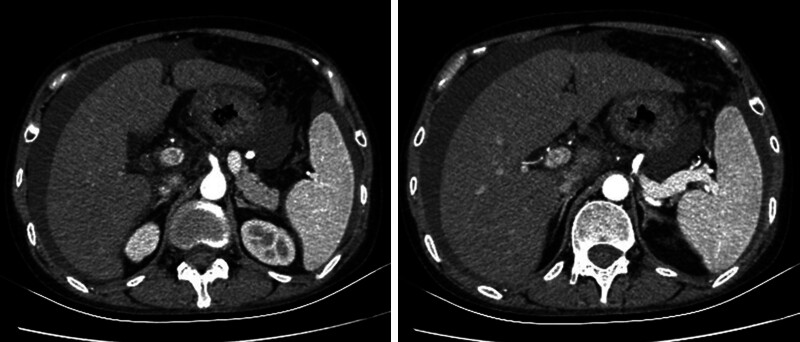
Contrast-enhanced abdominal CT showing ascites and portal vein dilatation without evident portal vein thrombosis. CT = computed tomography.
